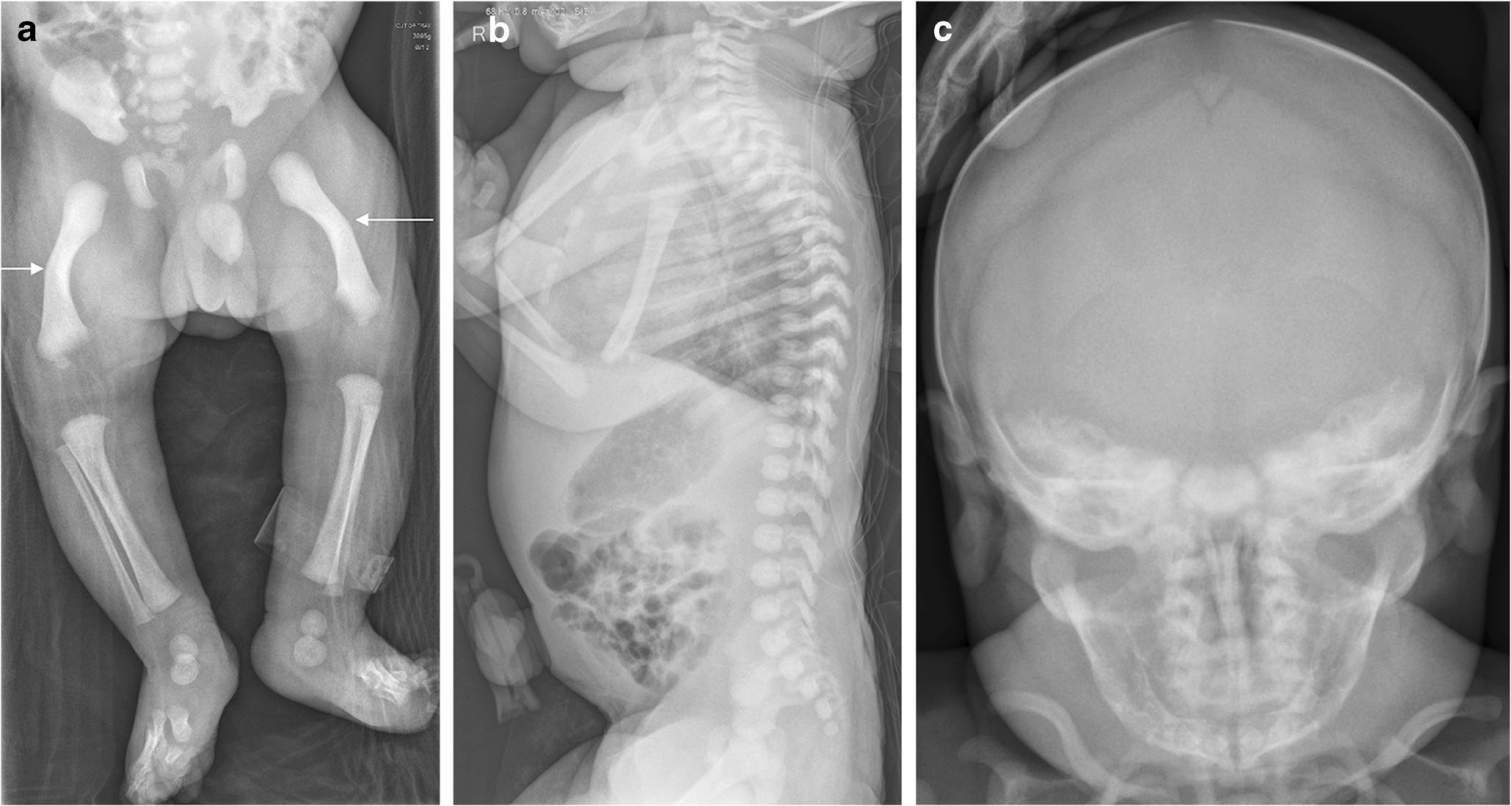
Images of a 1-day-old boy with a slowly progressing phenotype of perinatal hypophosphatasia. a Anteroposterior radiograph shows mild femoral bowing (*arrows*) of both lower limbs. b,c By comparison, the lateral spine (b) and anteroposterior skull (c) are relatively normal. The patient had low alkaline phosphatase activity and an elevated vitamin B6 concentration. Mutation of the *ALPL* gene was identified in the infant and mother. The child later had premature loss of primary dentition and femoral remodeling with growth
